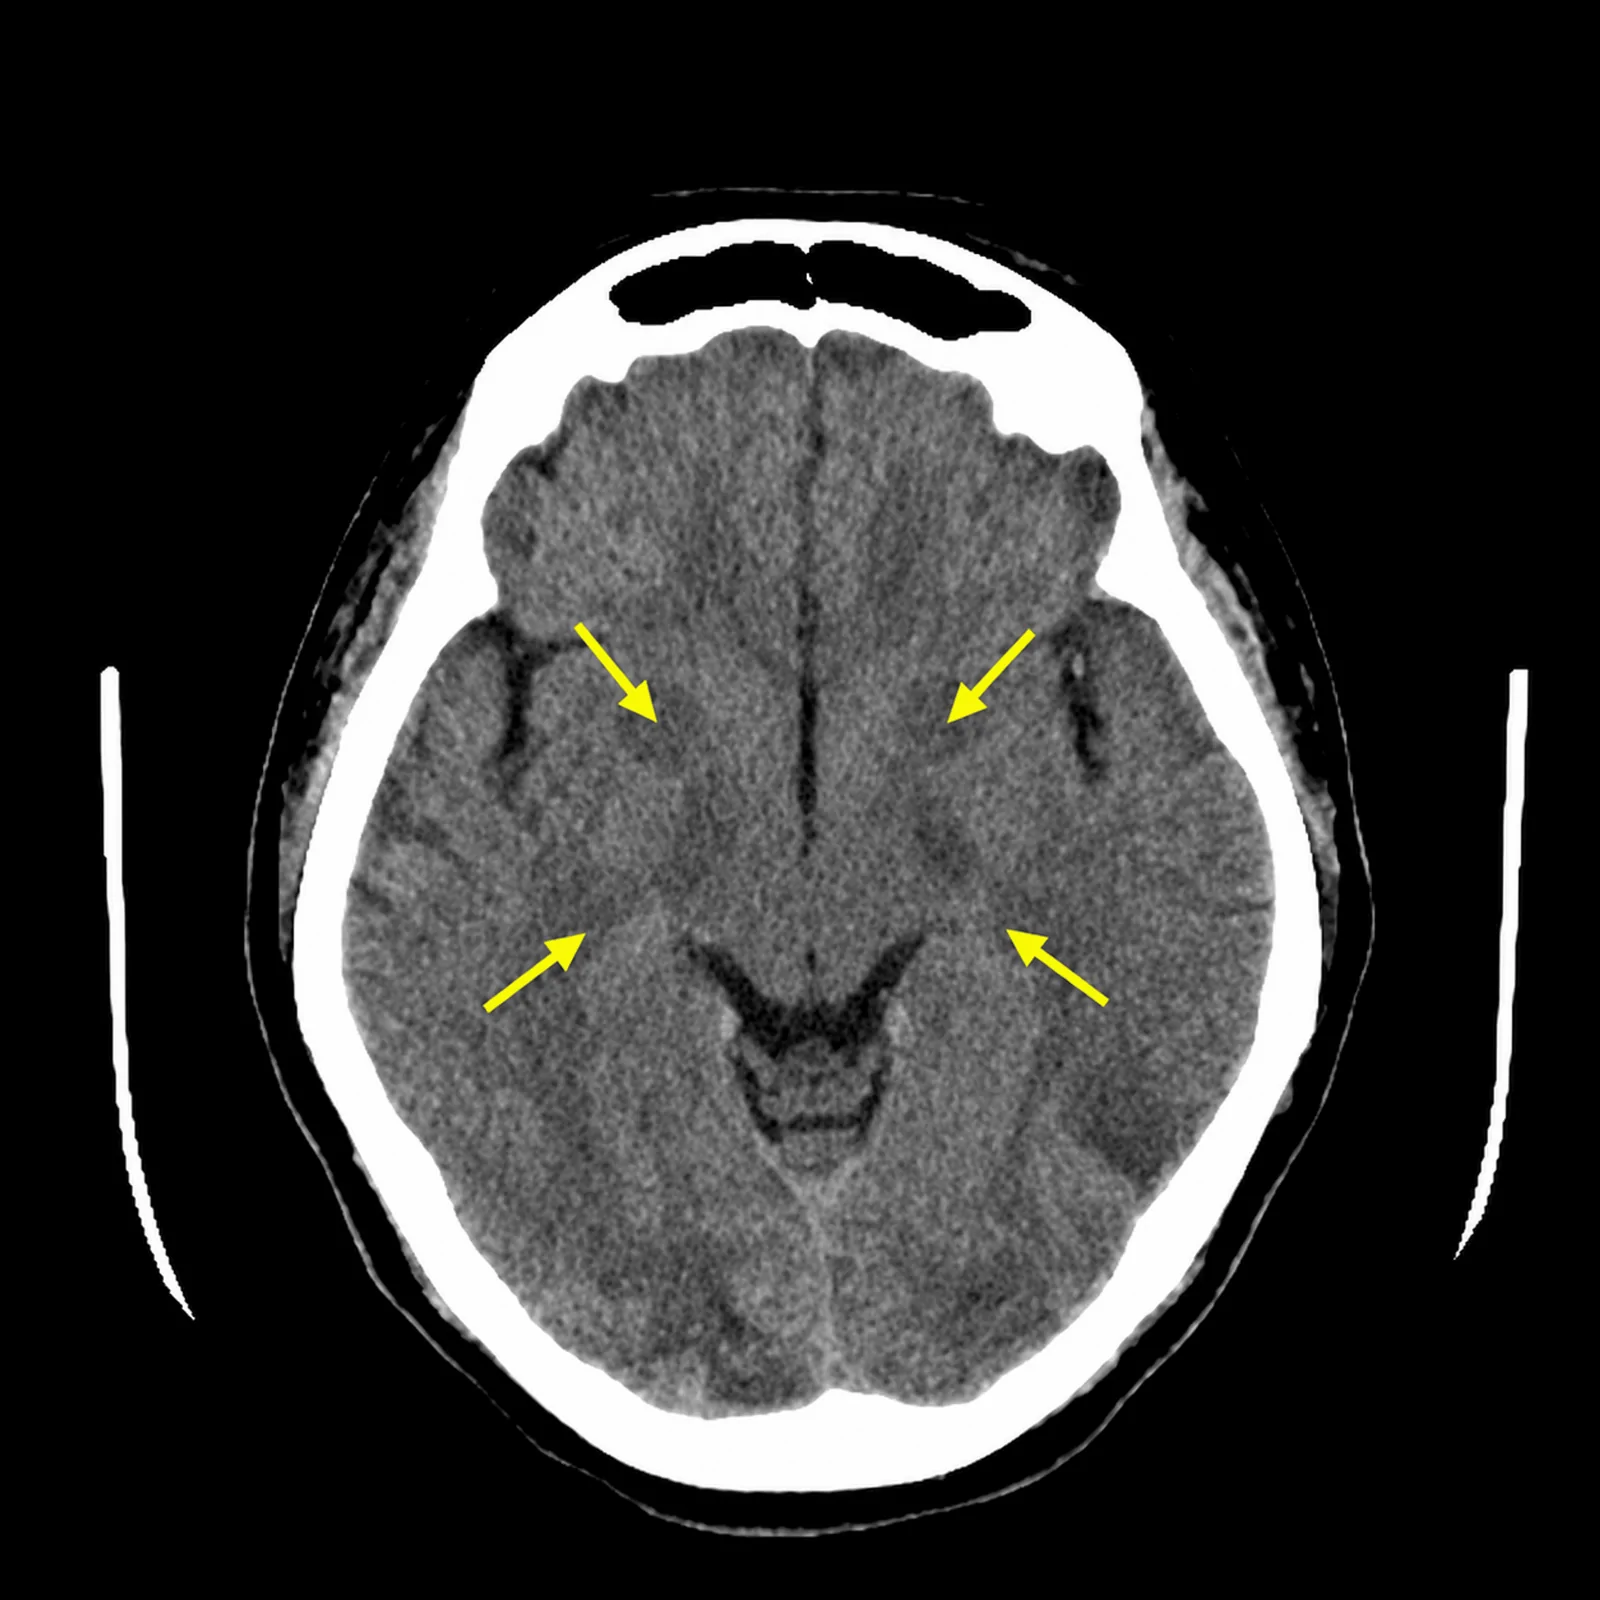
Axial non-contrast CT of the brain demonstrating hypoattenuation involving the caudate nuclei and posterior external capsules, representing the central component described in the radiology report. CT: computed tomography.
